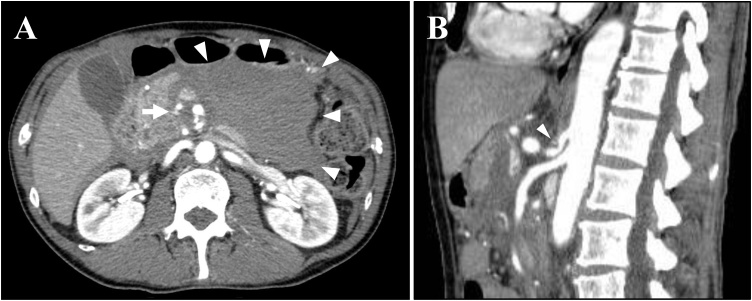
The findings of CT on admission: (A) Axial images showed a massive retroperitoneal hematoma around pancreas (white arrowheads) and a PDA aneurysm on pancreas head (white arrow). (B) Sagittal view of the CT angiography showed extrinsic compression of the root of the CA by the MAL (white arrowhead).
